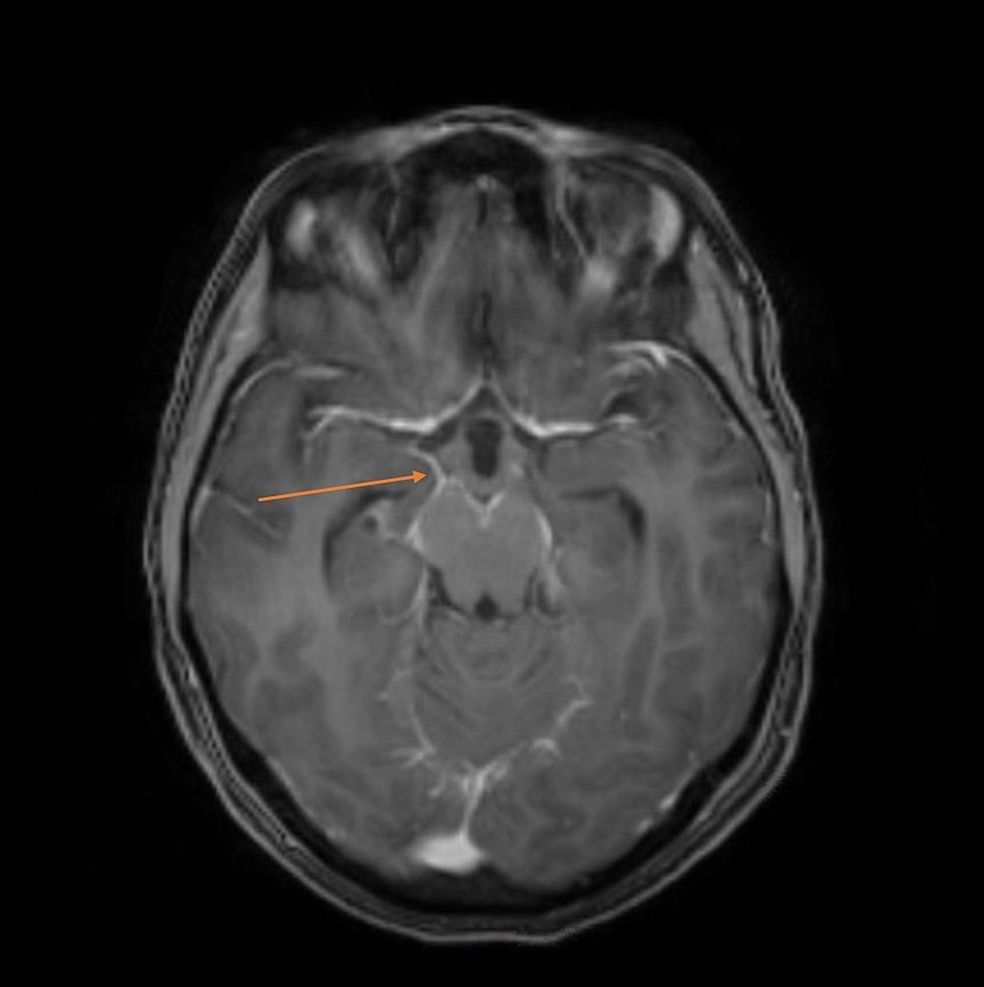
Contrast MRI of the brain demonstrating abnormal enhancement at the ventral brainstem lining the interpeduncular cistern, with temporal horns of the lateral ventricles visible, suggesting hydrocephalus MRI, Magnetic resonance imaging.
